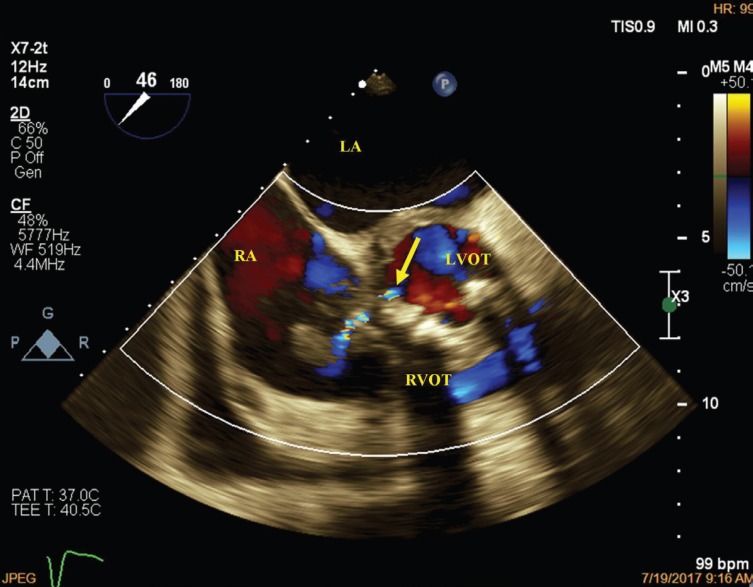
Transesophageal echocardiography in the 5-chamber view, demonstrating an aorto-mitral intervalvular fibrosa abscess rupturing to the LVOT (arrow).
